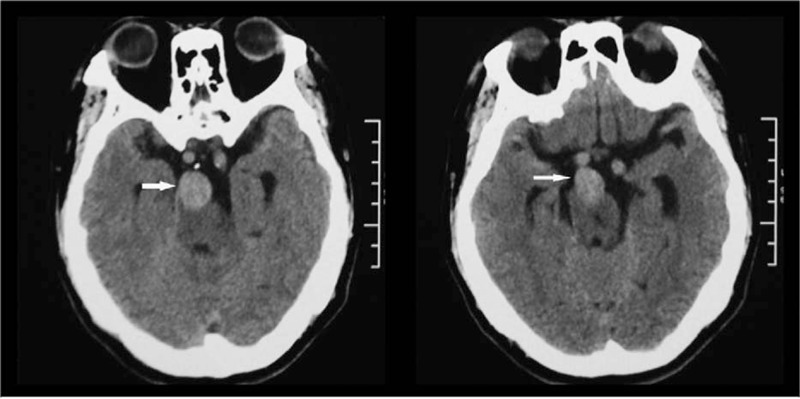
High-intensity signals on brain computed tomography.
